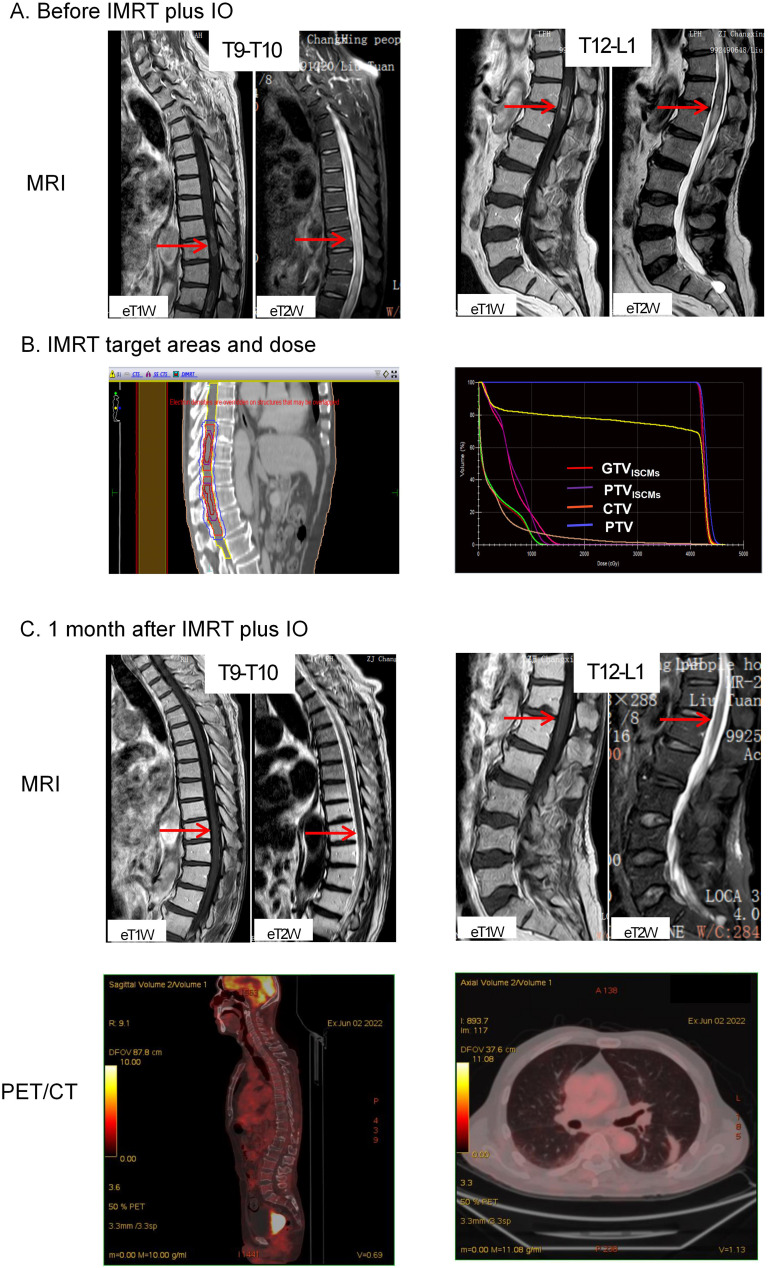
Imaging of tumor lesions before and after treatment. (A) MRI before IMRT plus IO showed that the signal of the spinal cord was not homogeneous, with foci of enhancement (indicated by red arrows) in the spinal cord at the T9-T10 and T12-L1 levels. (B) IMRT target areas in the median sagittal section (left) and total volume DVH (right). The bright red line is GTV_ISCMs_, the purple line is PTV_ISCMs_, the orange line is CTV and the blue line is PTV. The 100% radiation volume of GTV_ISCMs_, PTV_ISCMs_, CTV and PTV reached the dose of 40Gy, and the 95% radiation volume of PTV reached the dose of 42Gy. (C) MRI after IMRT plus IO treatment showed that the enhancement signal of the original lesions at T9-10 and T12-L1 levels decreased as an oedema signal (indicated by red arrows). In addition, PET/CT showed no abnormal FDG uptake throughout the spinal cord, mediastinum and both lungs. IMRT, intensity-modulated radiotherapy; IO, immunotherapy; MRI, magnetic resonance imaging; PET/CT, positron emission tomography/computed tomography; FDG, ^18^F-fluorodeoxyglucose. ISCMs, intramedullary spinal cord metastases; DVH, dose-volume histogram; GTV_ISCMs_, gross tumor volume of ISCMs; PTV_ISCMs_, planning target volume of ISCMs; CTV, clinical target volume; PTV, planning target volume.
